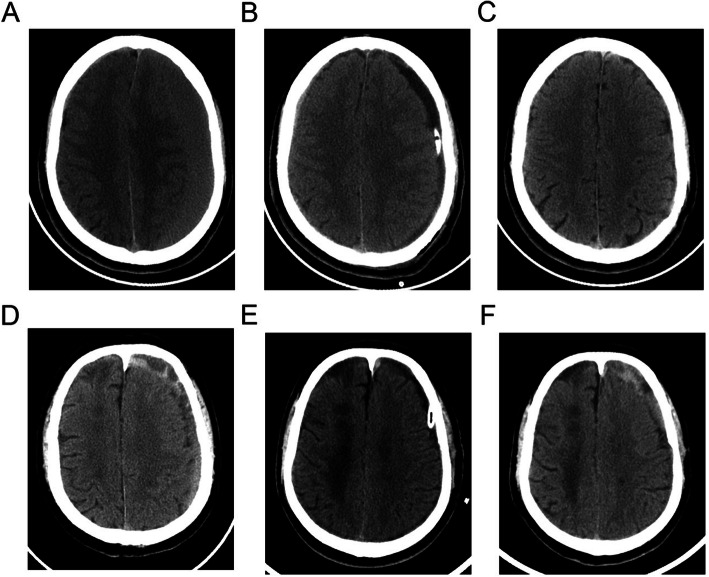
CT imagings of two typical patients without and with recurrence of CSDH. a, the preoperative presentation of patient A without recurrence; b the imaging of the same patient within 48 h postoperatively; c the CT scan at 3-month follow-up of the patient A; d the presurgical imaging of patient B with recurrence of CSDH; e the presentation of patient B within 48 h postoperatively; f the CT scan of patient B at 2 months after operation, a significant increase volume of subdural collection could be seen in left side
